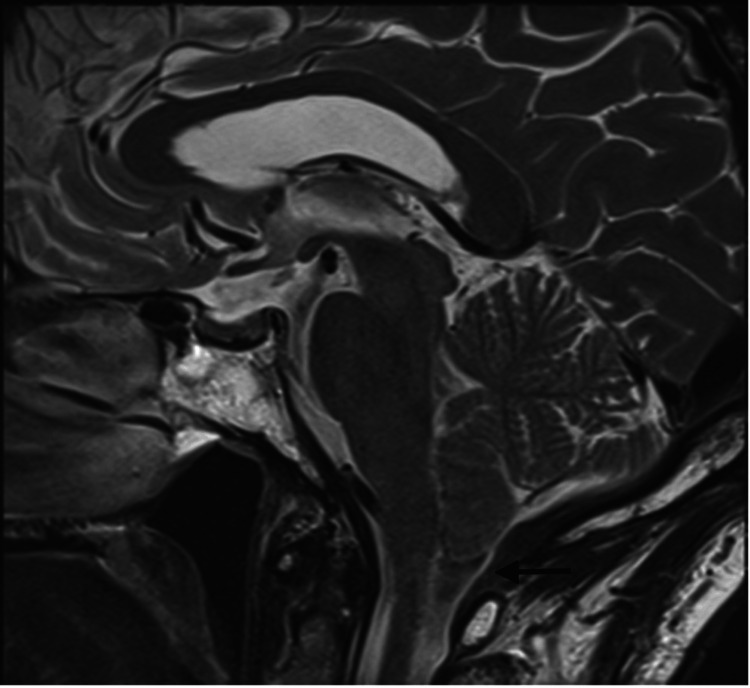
Sagittal T2 weighted spin echo MRI image of the midline brain demonstrating descent of the cerebellar tonsils 6 mm below the foramen magnum in keeping with Chiari type I malformation (arrow)
